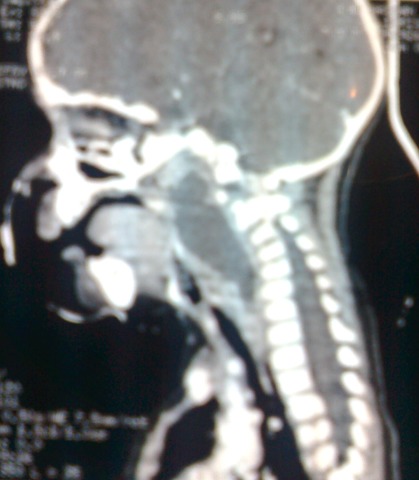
CT scan sagittal view that shows a retropharyngeal abscess with straightness of the cervical spine
